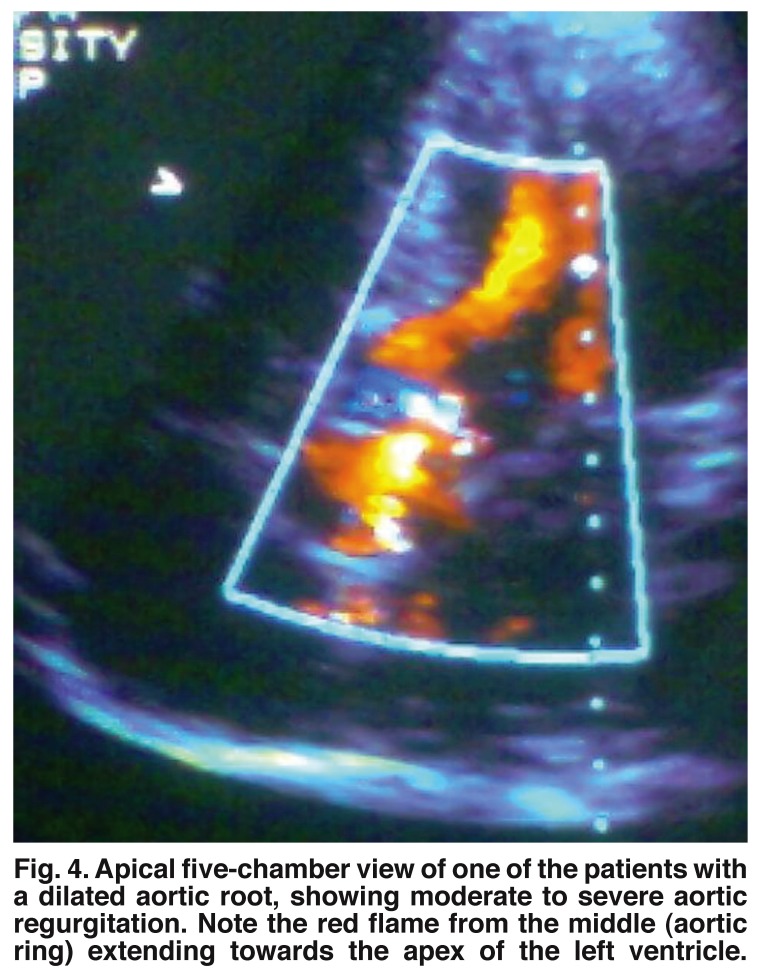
Apical five-chamber view of one of the patients with a dilated aortic root, showing moderate to severe aortic regurgitation. Note the red flame from the middle (aortic ring) extending towards the apex of the left ventricle.
